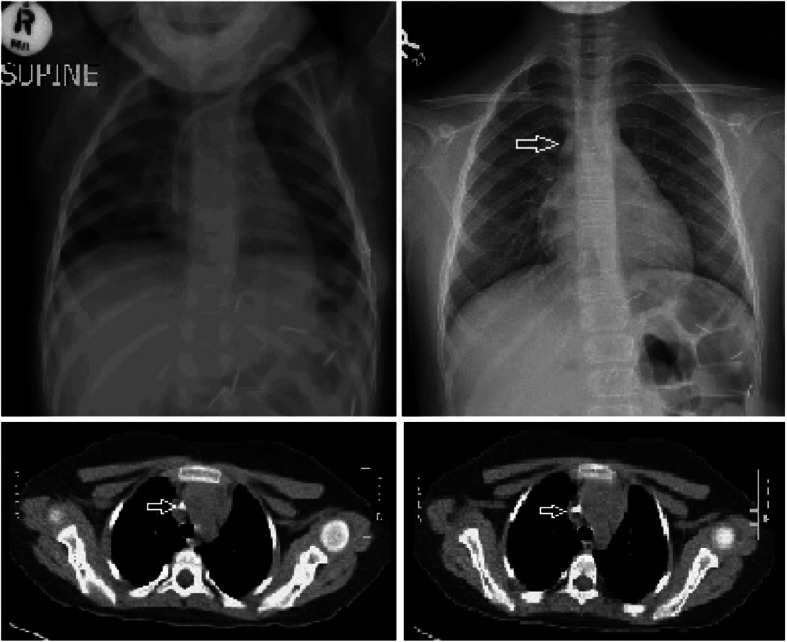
Follow-up chest X-ray and chest CT scan after 4 years of catheter removal showing the retained part of the catheter with no thrombosis of the superior vena cava
